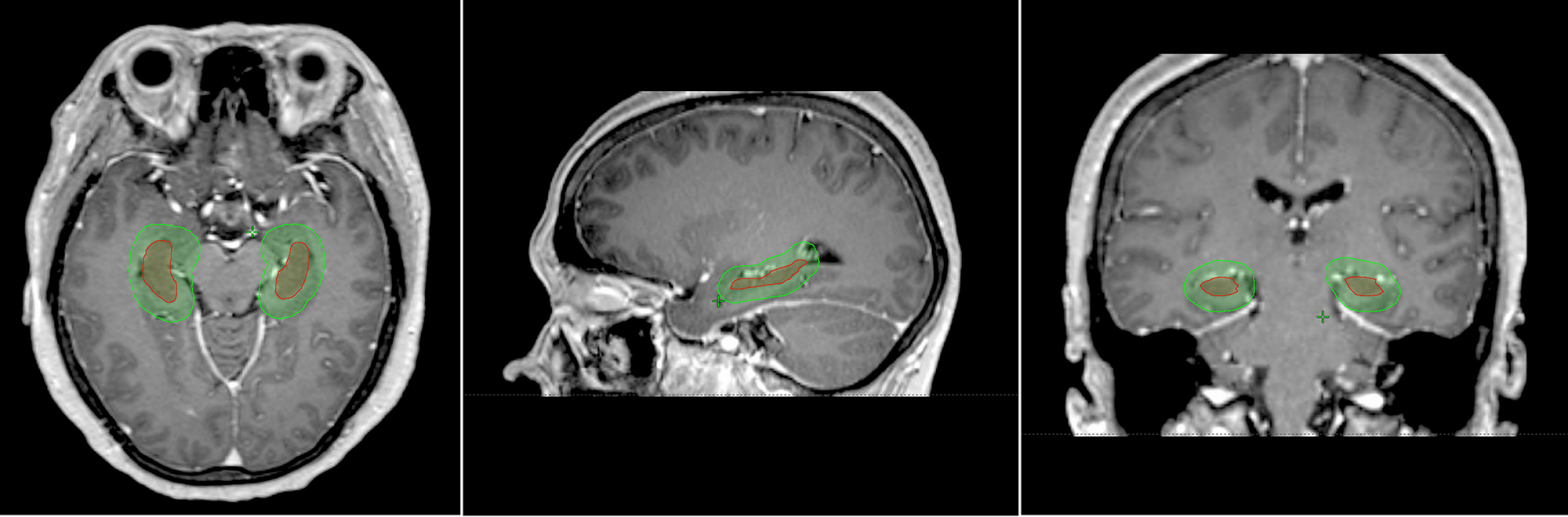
Hippocampus contoured on axial, sagittal, and coronal contrast-enhanced T1 magnetic resonance images The red contour showing the hippocampus contoured and the hippocampal avoidance region contoured in green.
